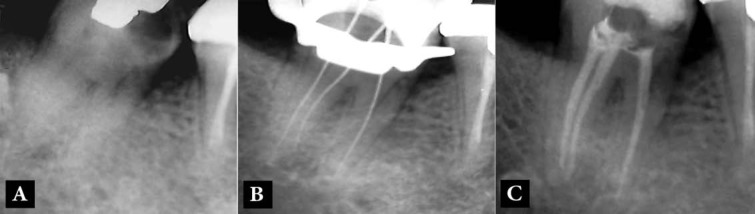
Case-2; *A)* Pre-operative radiograph shows the mandibular second molar with two separate roots; *B)* Working radiograph shows one mesial and two separate distal root canals; *C)* Post-operative radiograph; one mesial and two distal canals can be seen
